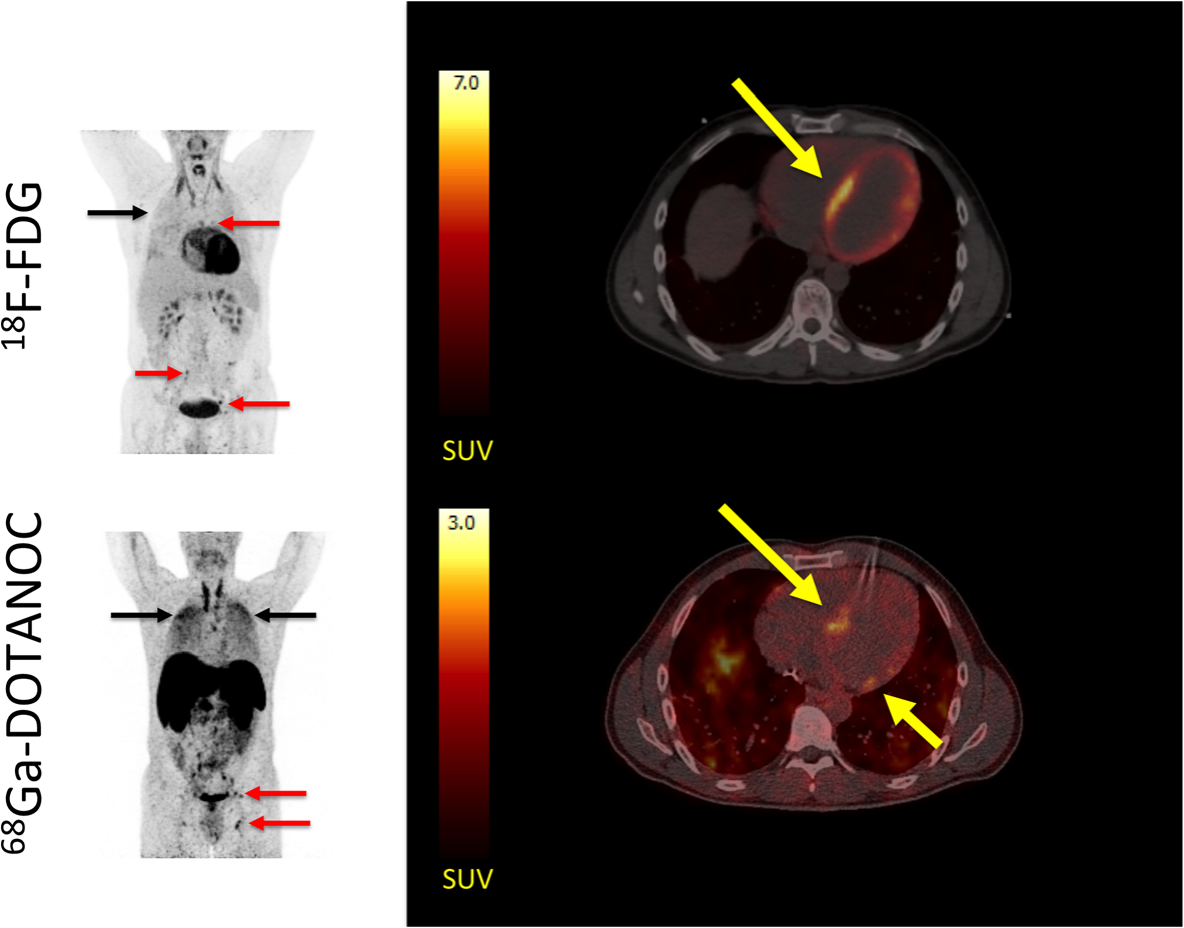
Patient with CS in which the ^18^F-FDG PET/CT was inconclusive due to insufficiently suppressed physiological ^18^F-FDG uptake by the myocardium. *Left panel*: MIPs showing patient no. 5 with dilated cardiomyopathy and multiple ^18^F-FDG and ^68^Ga-DOTANOC avid lymph nodes (*red arrows*) both above and below the diaphragm. In addition, there is massive and diffusely increased activity in the lung parenchyma (*black arrows*) representing active pulmonary sarcoidosis. *Right panel*: transaxial slices of the cardiac region reveal a focal on diffuse pattern of ^18^F-FDG uptake (*top*) raising suspicion of cardiac involvement (SUVmax 21 in the septum). However, the image was rated inconclusive by a majority of expert reviewers. By contrast, all reviewers rated the ^68^Ga-DOTANOC uptake (SUVmax 2.8, target-to-background 3.04) in the septum pathological (*bottom*). The patient was treated with corticosteroids and recovered
